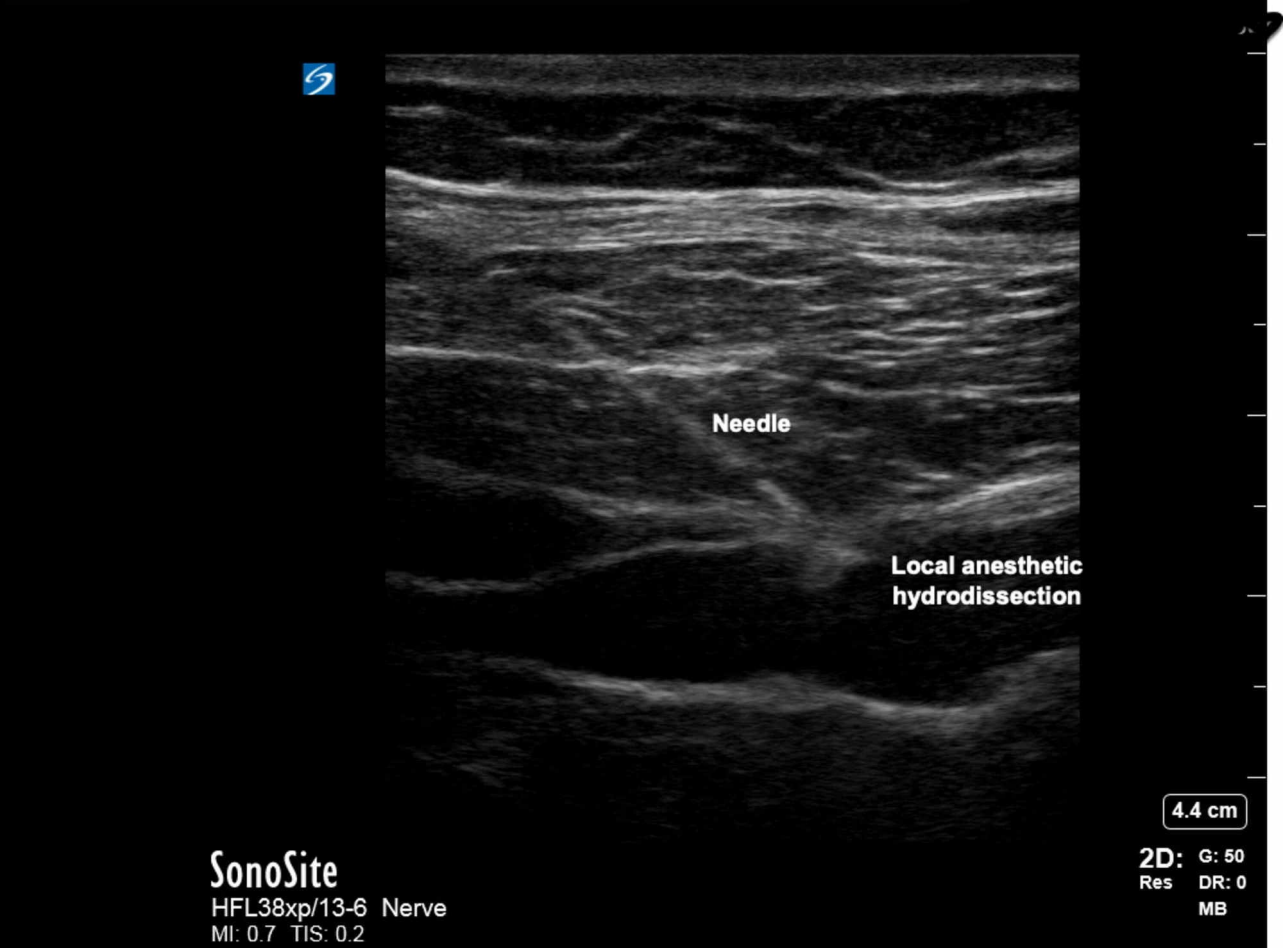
Ultrasound image of local anesthetic hydro-dissection for QL 2 block (QL2; posterior QLB). QL, quadratus lumborum; QLB, quadratus lumborum block
